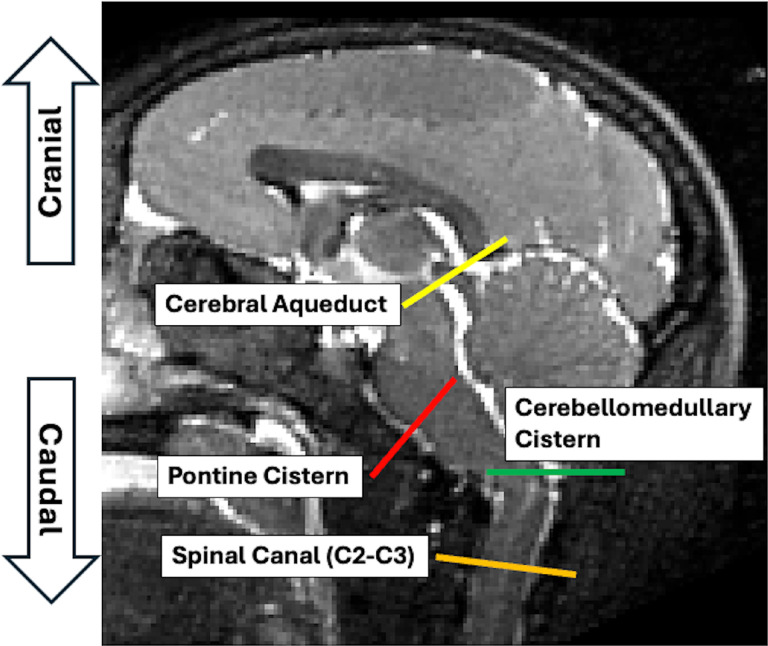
Imaging planes delineated on T2-weighted MRI and the corresponding phase contrast MR images, used for measuring CSF flow at specific regions of interest.
